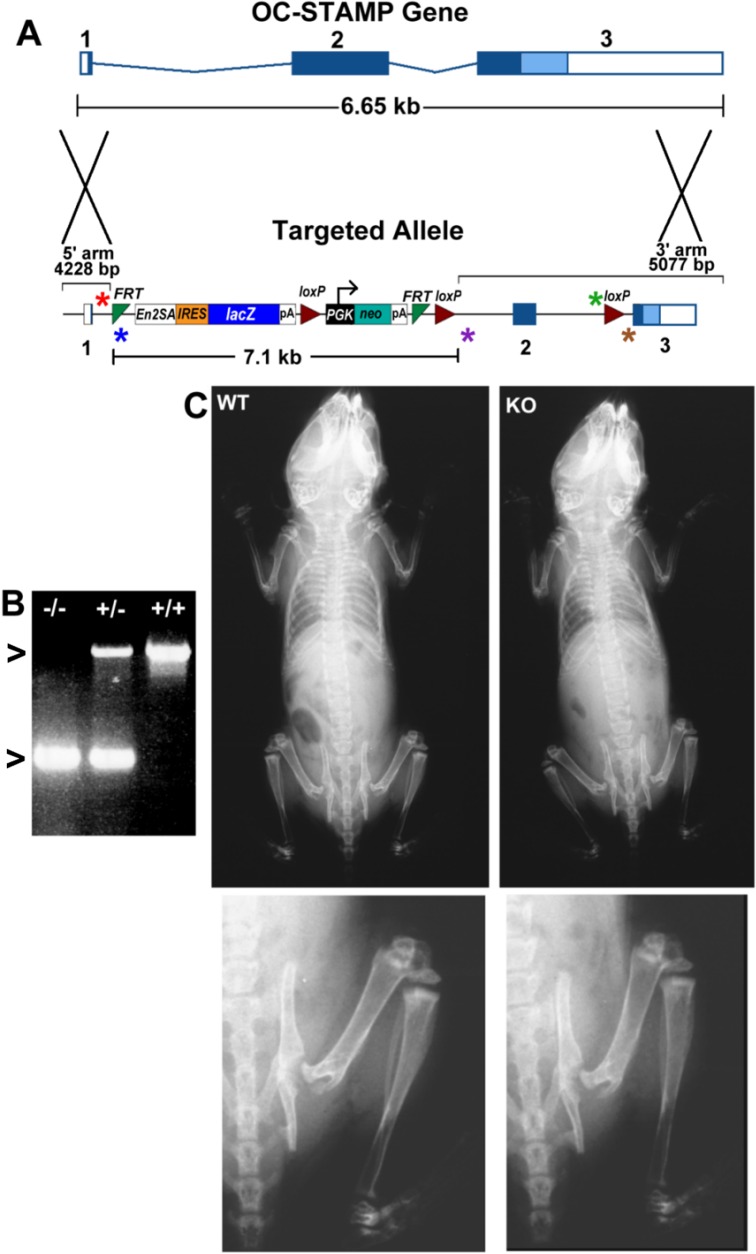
OC-STAMP knockout allele, radiographs. A. Gene targeting vector is a “knockout first” homologous recombination gene trap with a selection cassette and recombination sites for Cre (loxP) and flippase (Frt), as indicated. This allele, which results in a complete loss-of-function, is designated *Ocstamp*
